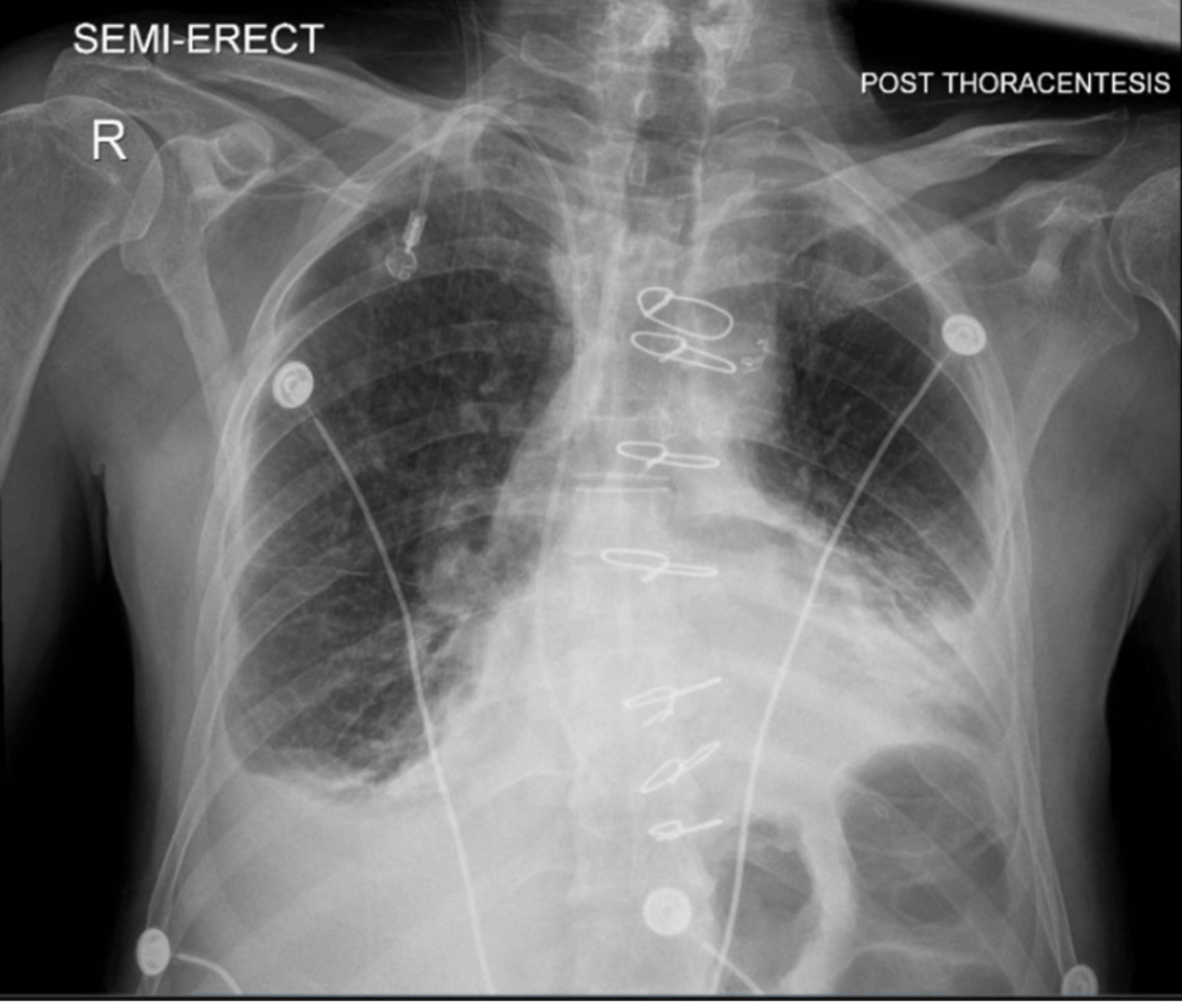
Post-thoracentesis single-view chest x-ray Lungs revealing reticular opacities. Ground-glass opacities throughout the right hemithorax. Presence of peripheral septal thickening and hilar vascular indistinctness. Pleura reveals obscuration over the right costophrenic angle and hemidiaphragm. Presence of hazy opacities overlying the left costophrenic angle and diaphragm. The cardio-mediastinal silhouette is enlarged. Mediastinal surgical clips. Seven intact median sternotomy wires. Findings are suggestive of pulmonary edema with small right-sided pleural effusion. No pneumothorax following thoracentesis.
